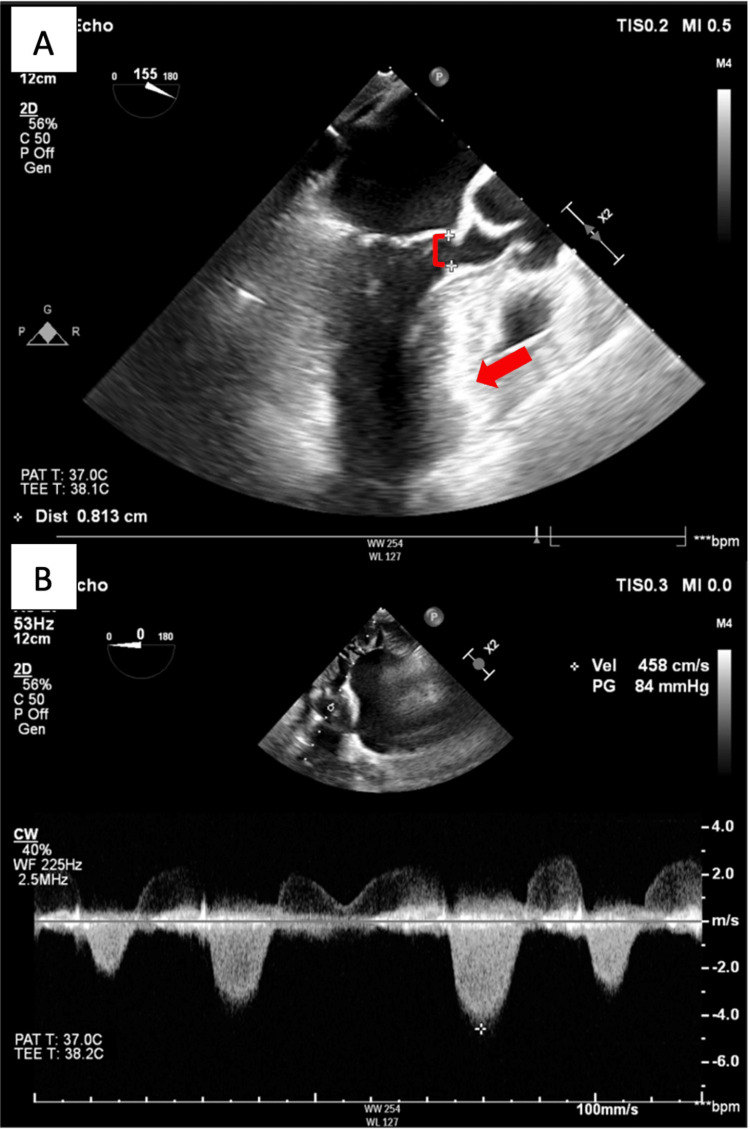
Intraoperative TEE demonstrating significant septal bulge and transvalvular systolic gradient across the AV A: Septal bulge (red arrow) with reduced systolic LVOT diameter (red bracket) at 0.81 cm (normal >2 cm);  B: Continuous wave Doppler across AV during systole. Subvalvular LVOT gradient at 84 mmHg (normal <30 mmHg) consistent with LVOT obstruction in the setting of septal HOCM. The interventricular septum diameter was 2 cm (not measured on the image). TEE: Transesophageal echocardiogram, LVOT: Left ventricular outflow tract, AV: Aortic valve, HOCM: Hypertrophic obstructive cardiomyopathy
